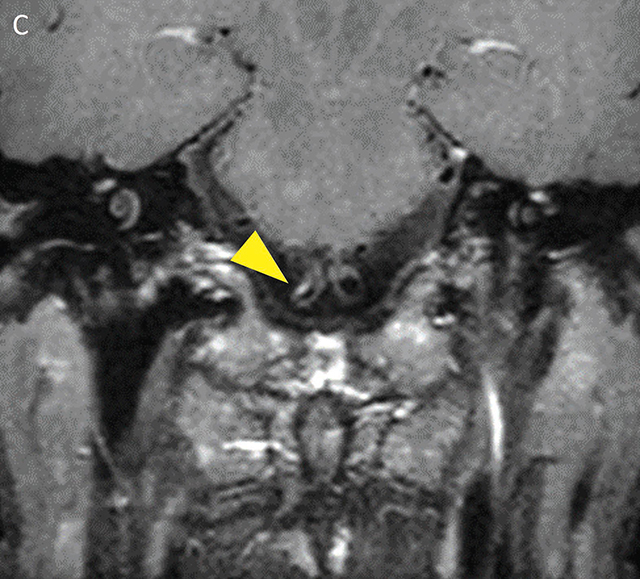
Black-blood-MRI showing focal intraluminal contrast enhancement of the V4 segment of the right vertebral artery in the coronal plan (yellow arrowhead).
